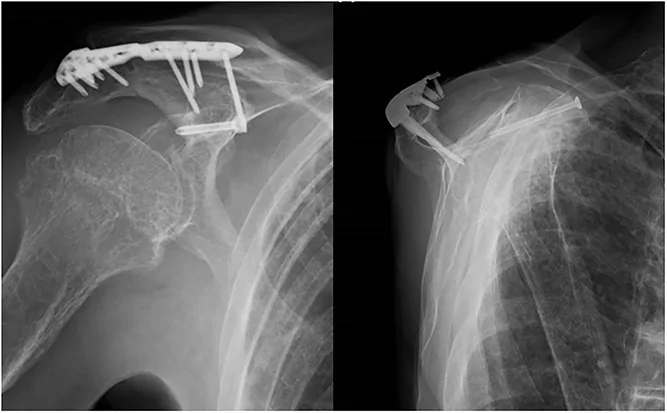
Postoperative radiographs of the right scapula obtained 1 year after surgery.
